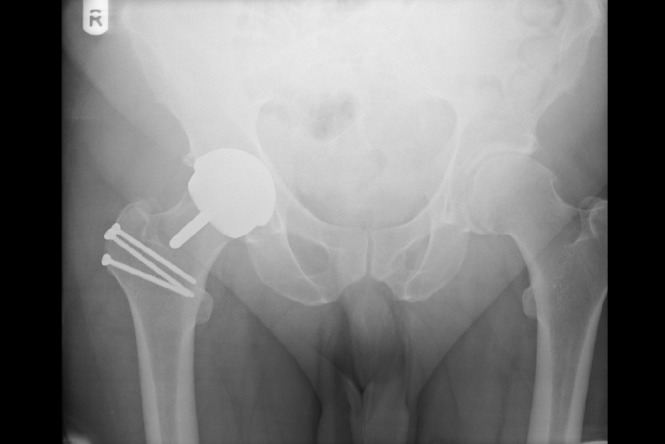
Post-operative x-ray following hip resurfacing
